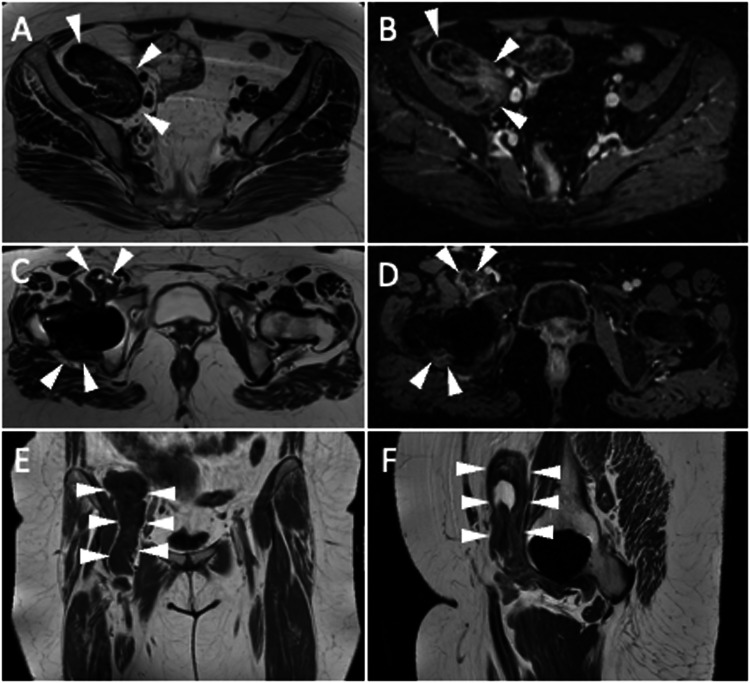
Pelvis MRI of a 58-year-old female who underwent prosthetic replacement of the right hip due to intra-articular tenosynovial giant cell tumor and experienced relapse of disease. Unenhanced axial T2-weighted (A, C), post-contrast fat-suppressed 3D GRE T1-weighted (B, D), unenhanced coronal T1-weighted (E), and unenhanced sagittal T2-weighted (F) images show periprosthetic tissue (arrowheads), located around THA neck and within the iliopsoas bursa, with T1- and T2-hypointensity and strong enhancement, similar to pseudotumor
